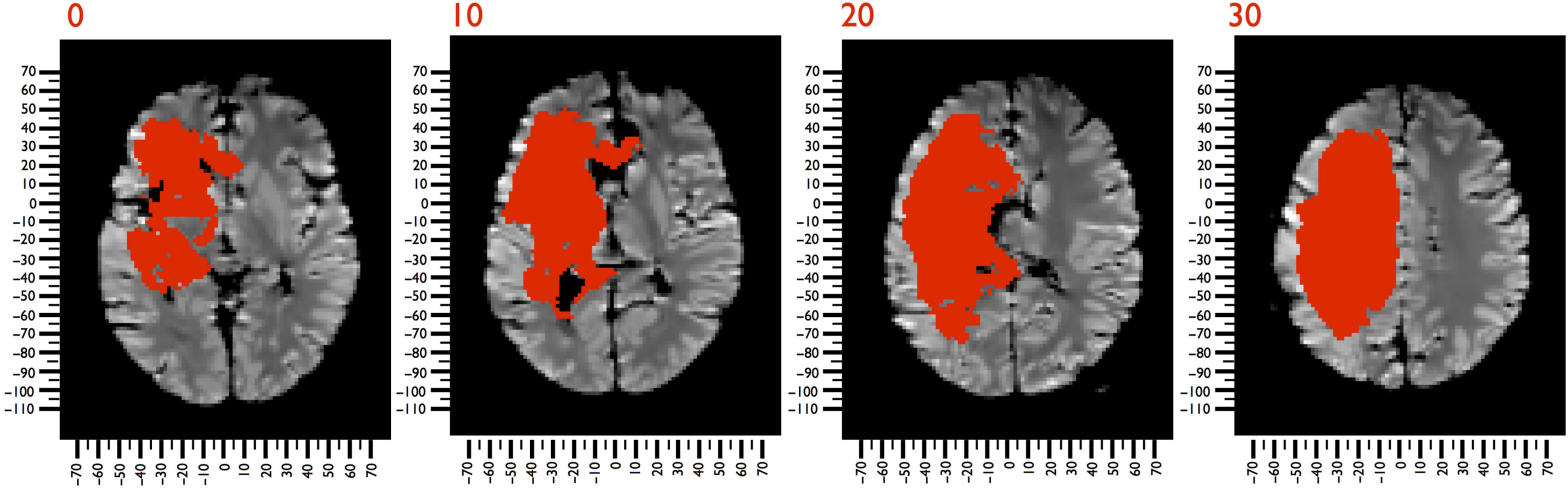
T*_1_-weighted MRI images of Leborgne's brain, registered to MNI152 space. The damage produced by the stroke is indicated in red.
